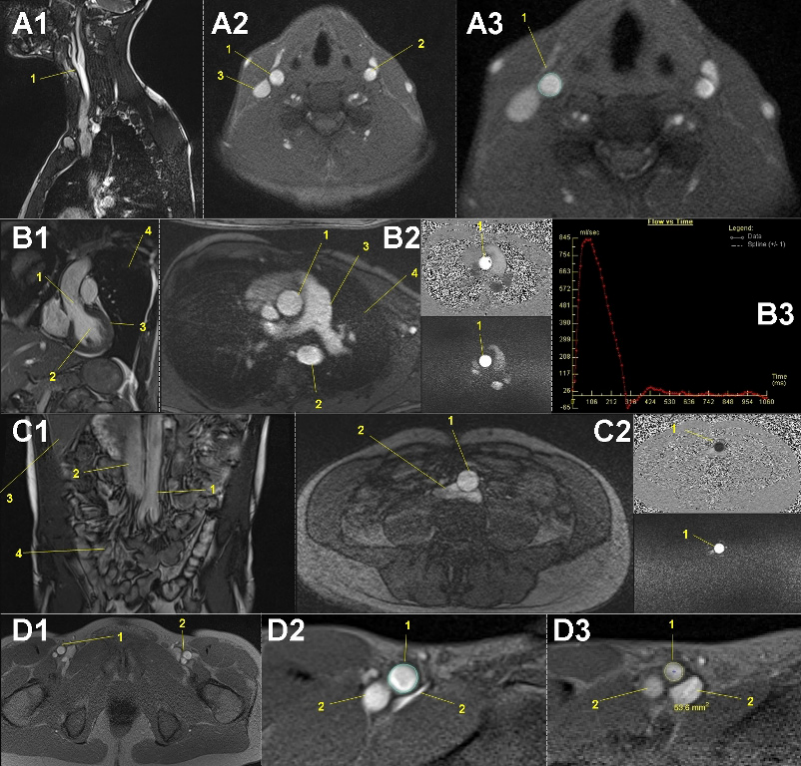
Mobile vascular cine-MRI: male, 52-years-old, stage 27, 1,838 km. A1: MR localizer sagittal, A2,3: FLASH transversal: Automatic functional measurement of common right carotid artery diameter just below carotid bifurcation (1). Left carotid artery (2), right deep jugular vein (3). B1: MR localizer, B2: phase contrast transversal: Ascending aortic (1) diameter and flow is measured by specific velocity-encoded (venc) MR imaging. Descending aorta (2), pulmonary artery (3), lung (4). B3: graphic depiction of aortic pulsatile flow (ml/second). C1: MR localizer coronal, C2: phase contrast transversal: Distal descending aortic (1) diameter and flow is measured by specific velocity-encoded (venc) MR imaging just above the aortic bifurcation. Inferior vena cava (2), liver (3), intestines (4). D1: FLASH transversal: Functional measurement of superficial right femoral artery diameter just below bifurcation (1), left femoral artery (2). D2: Manual diameter measurement (1), D3: Automatic diameter measurement (1). Femoral veins (2).
